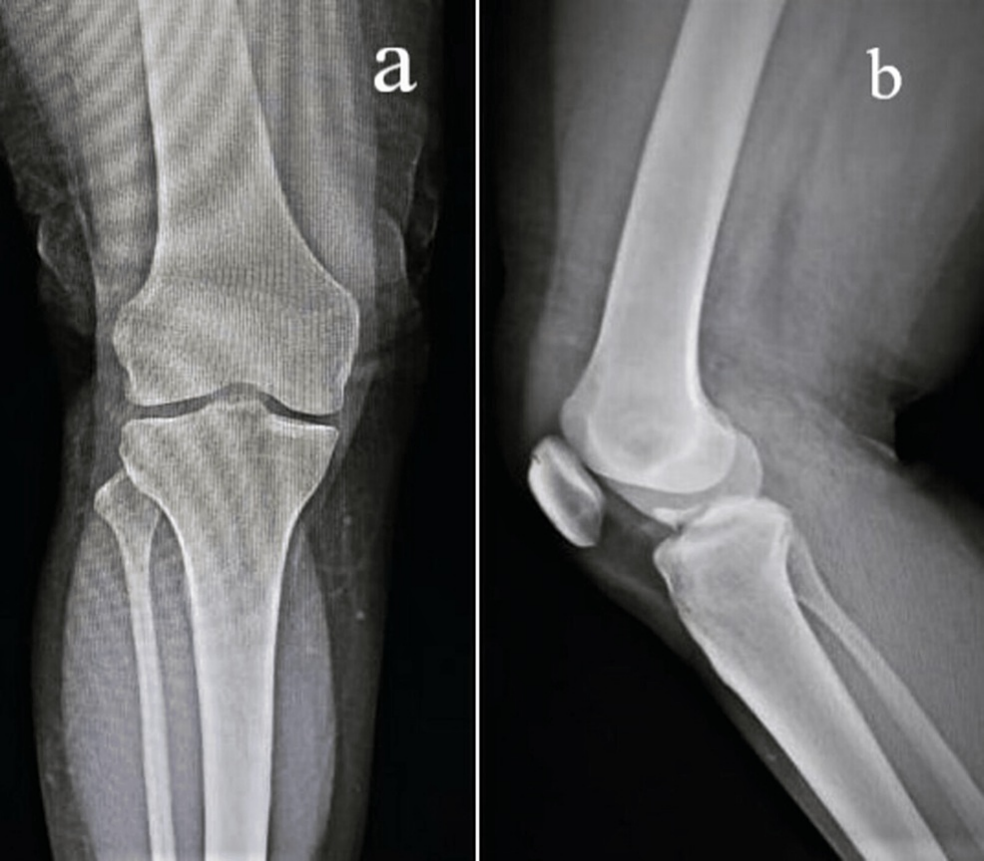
Radiograph of the left knee in AP (a) and lateral (b) views with maintained joint space, and articular cartilage.
